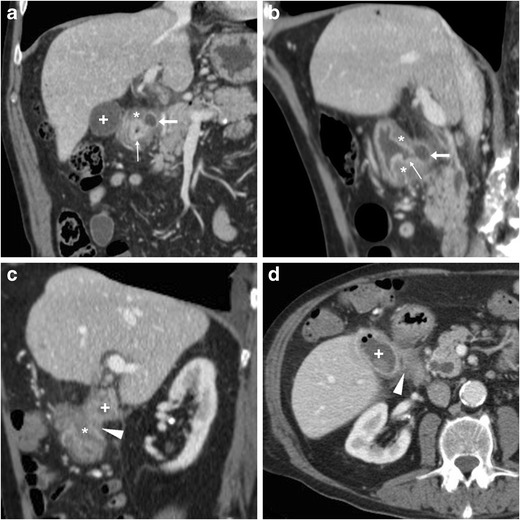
A 78-year-old male with history of chronic NSAID use had initial contrast-enhanced CT (a, b) finding of circumferential thickening of pylorus and proximal duodenum with oedematous submucosa (*), enhancing mucosa (thin arrows) and posterior ulcer outpouching (arrows); ventrally, the normal-appearing gallbladder (+) was in contact with the affected duodenal bulb. Fifteen months later, the patient experienced melaena and abdominal pain: repeated CT (c, d) showed development of communication (arrowheads) between thickened duodenum (*) and contracted gallbladder (+) with intraluminal air and some dependent sludge or blood
